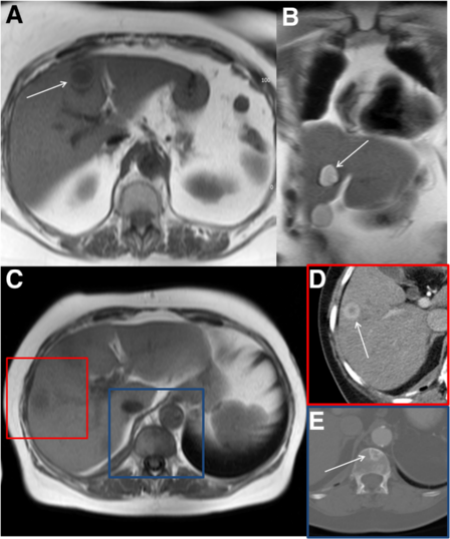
Axial black blood imaging (a) and coronal bright blood imaging (b) showing a well-defined low and high signal entity respectively consistent with a simple liver cyst. Axial black blood imaging (c) showing ill-defined low signal entity in the right lobe of liver (red box) which demonstrated features consistent with a metastasis on contrast enhanced CT (d). There is also a low signal entity in the vertebral body (blue box) which was confirmed to represent a sclerotic metastasis on CT (e)
